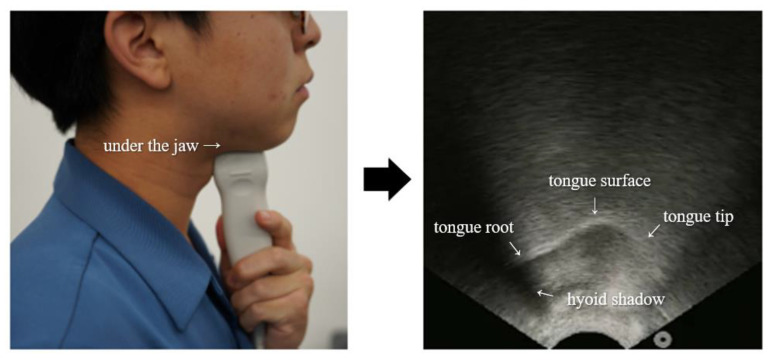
SottoVoce based on ultrasonic image [100].
